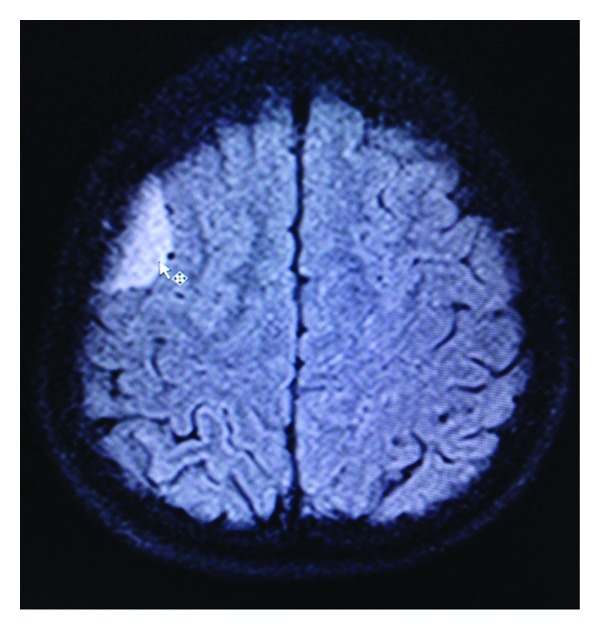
Axial fluid attenuated inversion recovery MR image showing broad dural based extra axial en plaque meningiomas at right frontoparietal region.
